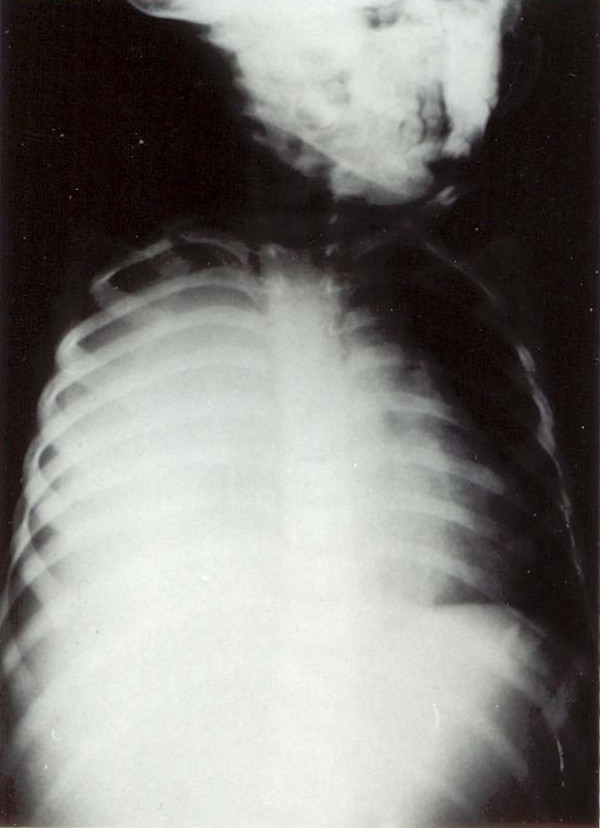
Chest X-ray showing right-sided massive pleural effusion. (A) Before drainage. (B) After drainage.
